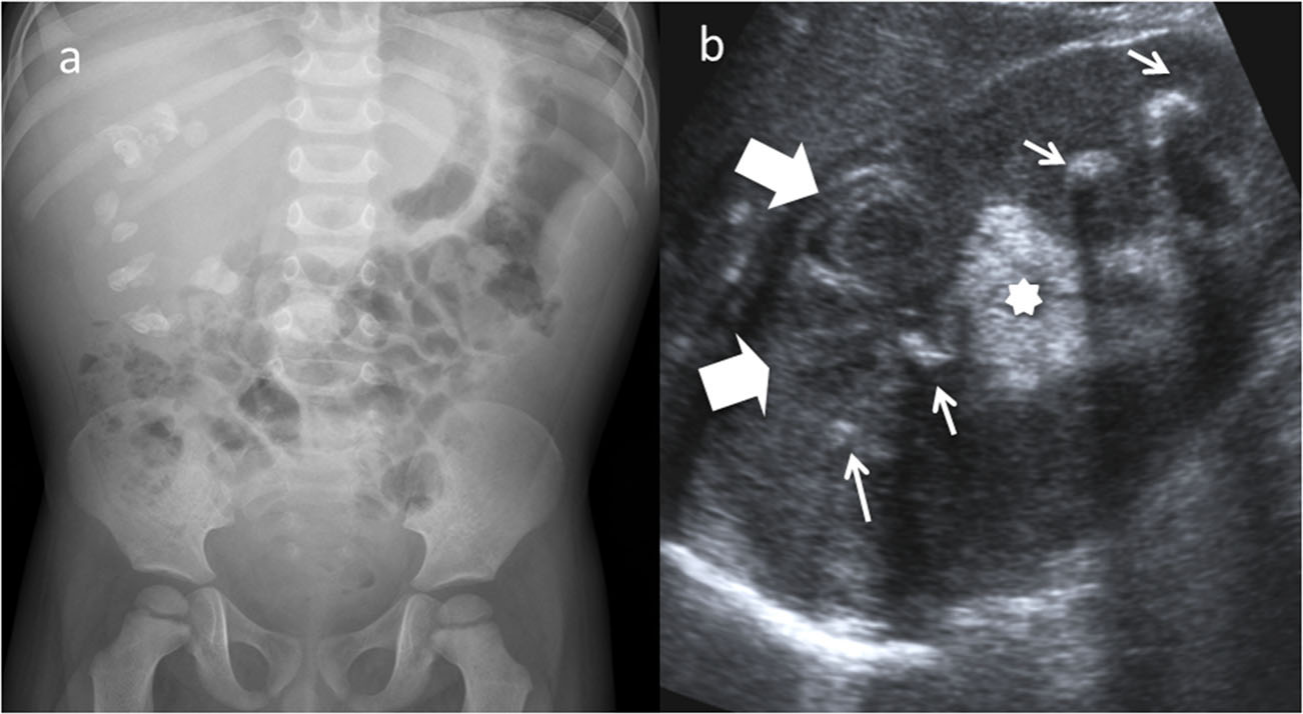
Diffuse XPN. a Plain X-ray of the abdomen showing multiple calculus in right kidney. b Longitudinal ultrasound shows calculus (*narrow white arrows*) in a large kidney with peripheric hypoechoic areas (*thick white arrows*) and inflammatory exudate (*asterisk*)
